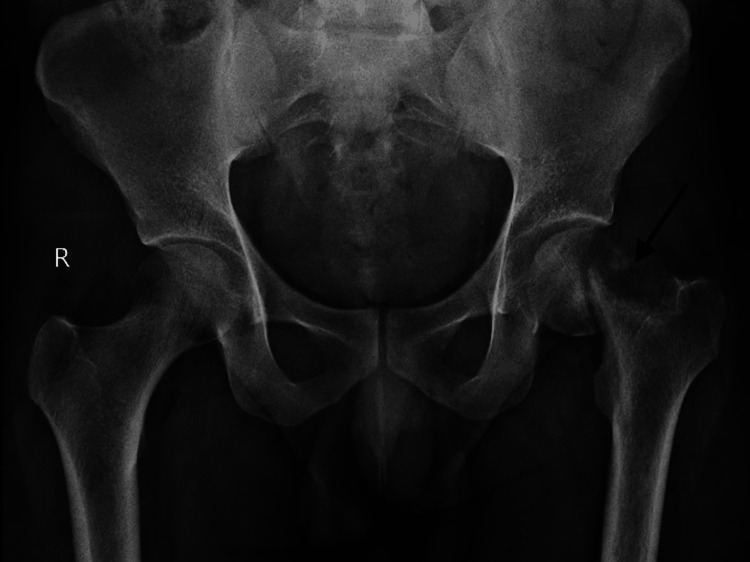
X-ray of the pelvis with both hips showing a sub-capital femoral neck fracture on the left side
